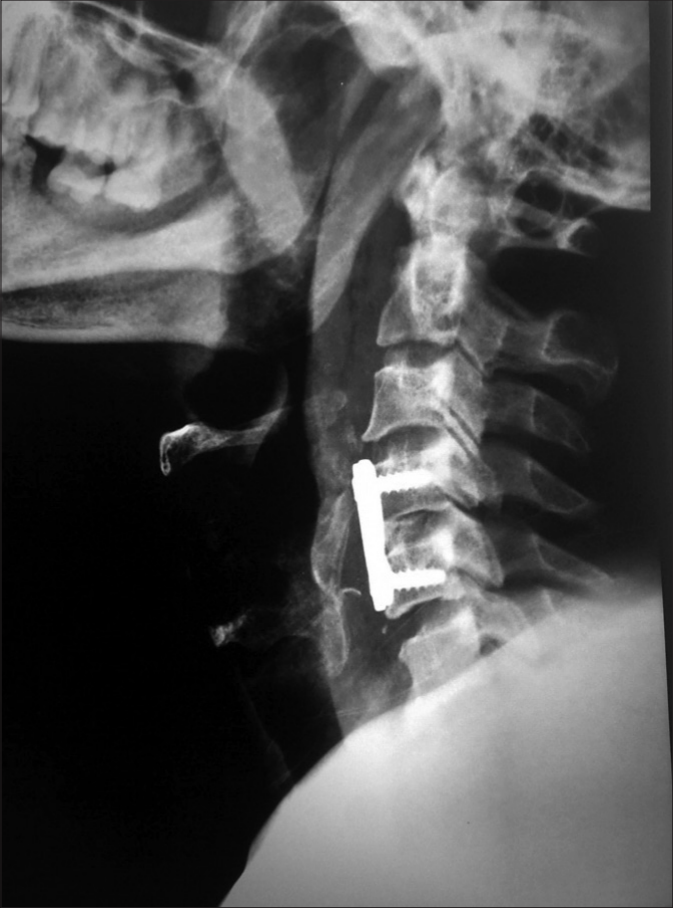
Follow up radiograph of cervical spine (lateral view) of a case of C4-C5 retrolisthesis showing good fusion and alignment obtained by anterior decompression, fixation, and fusion
